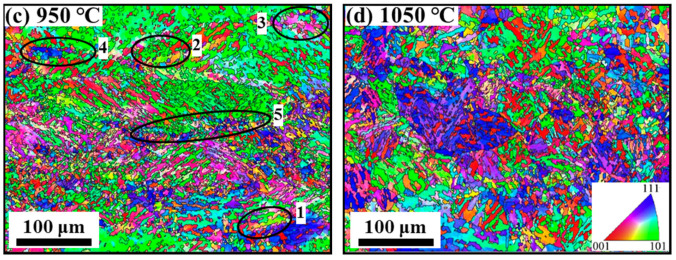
IPF maps of samples deformed at 0.1 s^−1^ at different deformation temperatures of (a) without ultrasonic 950 °C, (b) without ultrasonic 1050 °C, (c) with ultrasonic 950 °C, (d) with ultrasonic 1050 °C.
